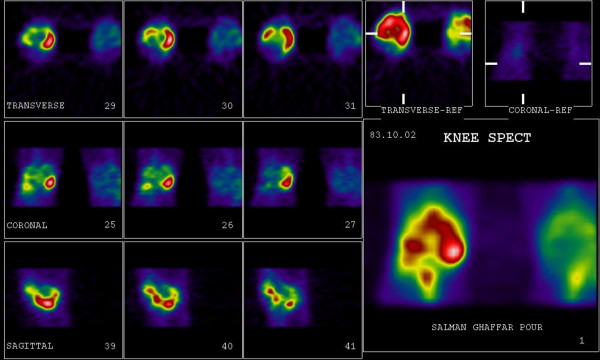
SPECT images of the same patient (presented in Figure 1.) showing a crescent of increased activity in the medial tibial plateau, which is scintigraphically characteristic feature of a meniscal tear.
